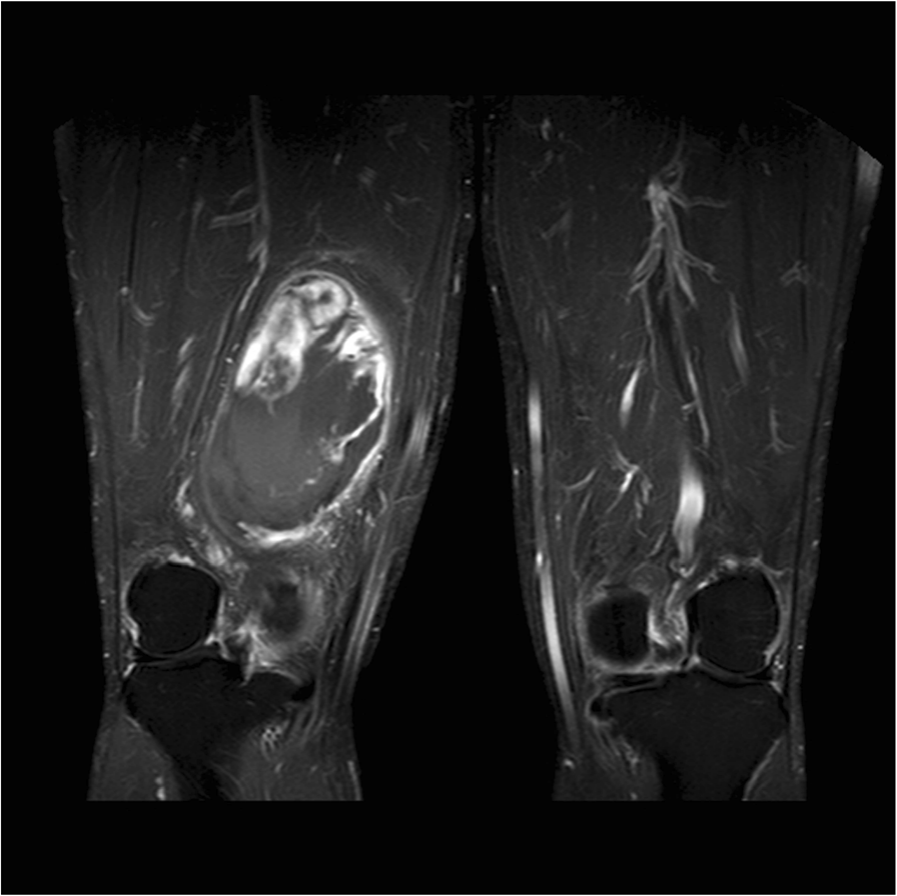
Magnetic resonance T2-weighted image on lower extremity
